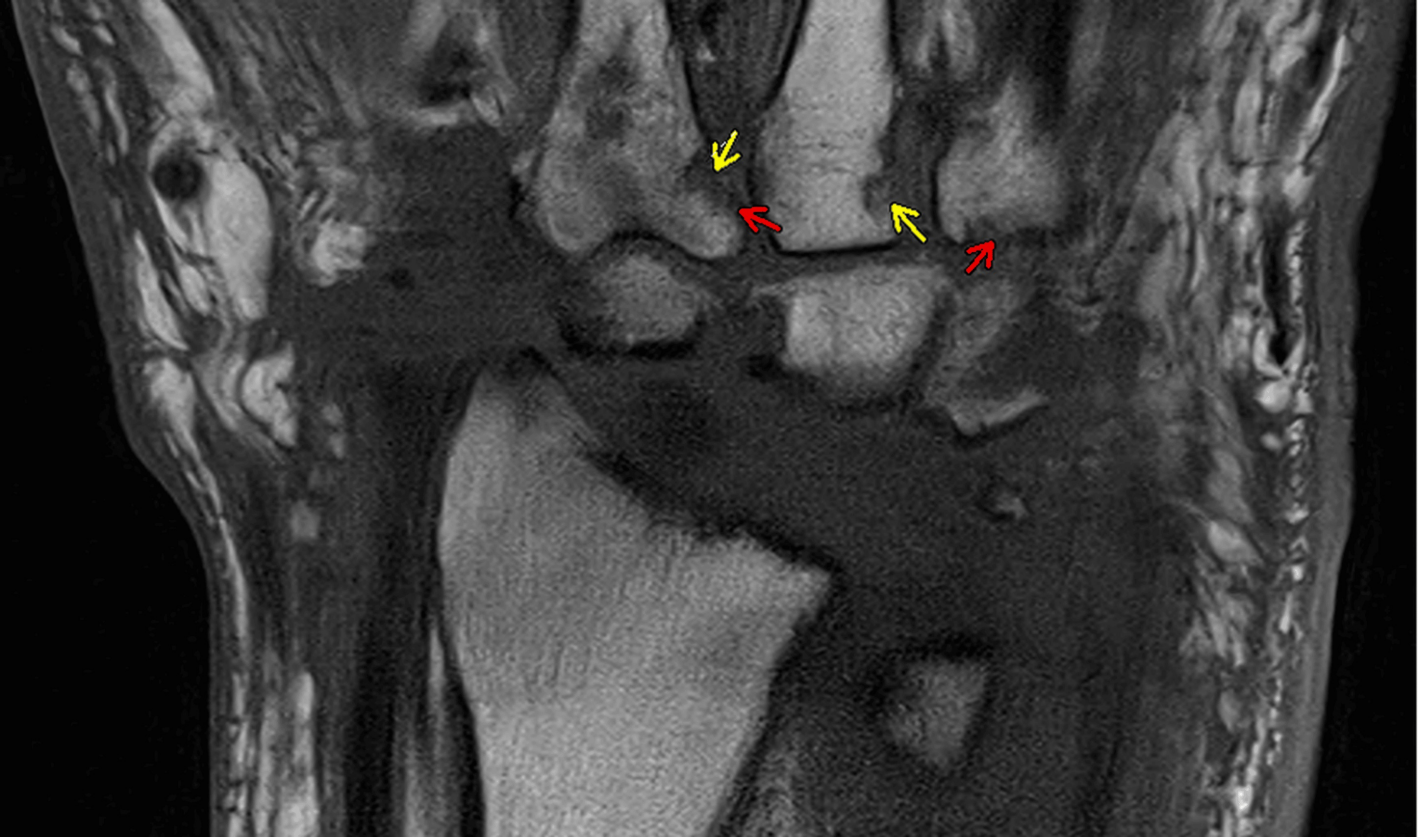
Coronal MRI of the left wrist demonstrating pancarpal joint effusion and synovial thickening (red arrows), along with subchondral cystic changes and erosions (yellow arrows), consistent with chronic inflammatory arthropathy and CPPD flare rather than acute septic arthritis CPPD: calcium pyrophosphate deposition
